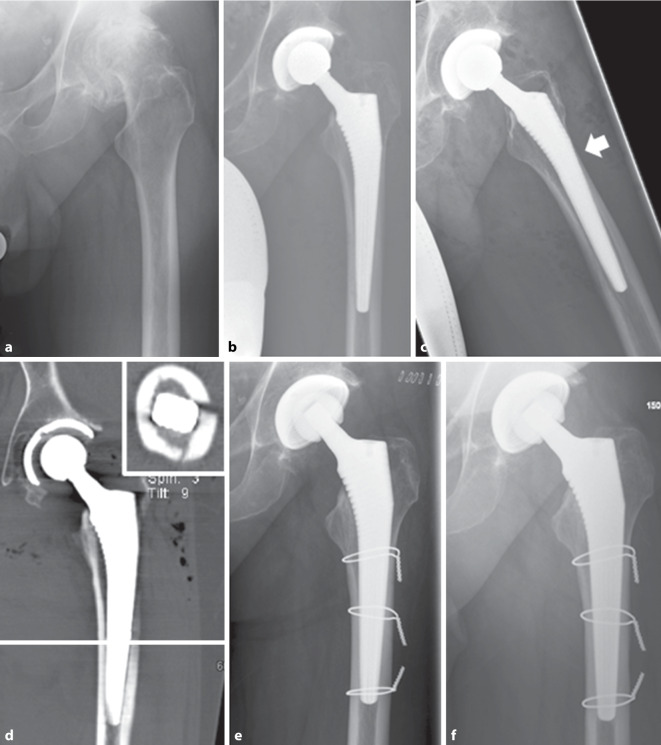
a 58 Jahre, männlich. Fortgeschrittene destruierende Koxarthrose. b/c Postoperative Röntgenkontrolle a. p. und axial nach Lauenstein mit dem V. a. eine Sinterung bei periprothetischer Femurfraktur (PPF, *Pfeil*) unmittelbar postoperativ. d Postoperatives CT (koronar und axial; die *weiße Linie* entspricht der Höhe in der axialen Projektion) mit Bestätigung des gesinterten Schaftes (PPF Vancouver-Typ B2). e Postoperative Kontrolle nach Revisionsoperation mit offener Reposition und Osteosynthese (1,5 mm Drahtcerclagen) sowie zementfreiem Schaftwechsel. Sicherungscerclage auf Höhe der Schaftspitze mit ausreichend Abstand zur anatomisch reponierten und fixierten Fraktur. Postoperativ 6 Wochen Entlastung der linken unteren Extremität. f In der Nachuntersuchung 1 Jahr nach der Revision zeigt sich eine konsolidierte Fraktur bei unveränderter Schaftlage
